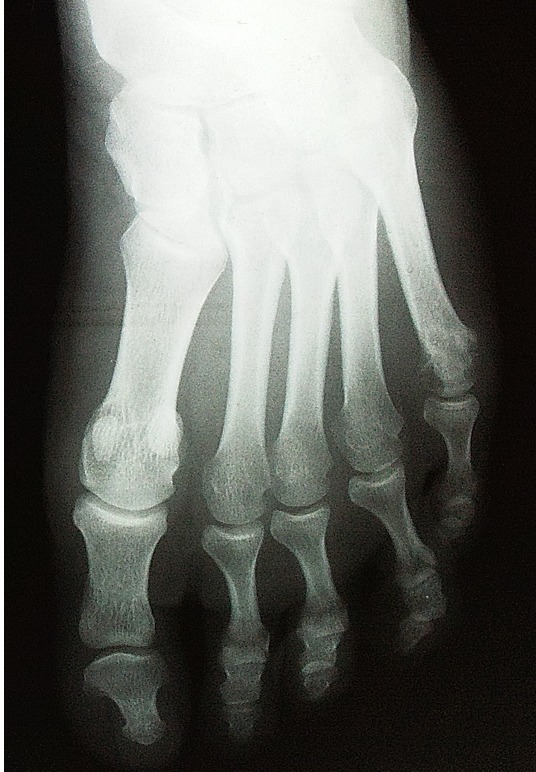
Radiographie du pied gauche face: géodes de la tête du 5ème métatarsien gauche
